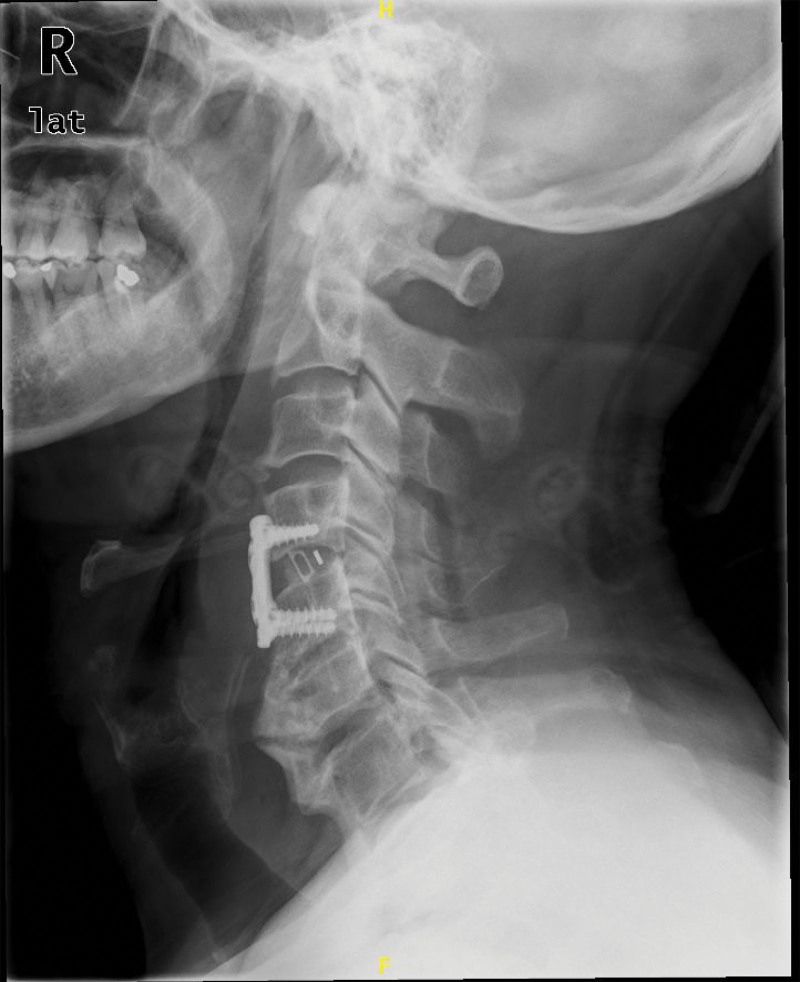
Immediate postoperative lateral cervical X-ray showing the implanted C4 and C5 interbody cage and anterior cervical plate with screws in satisfactory position.
